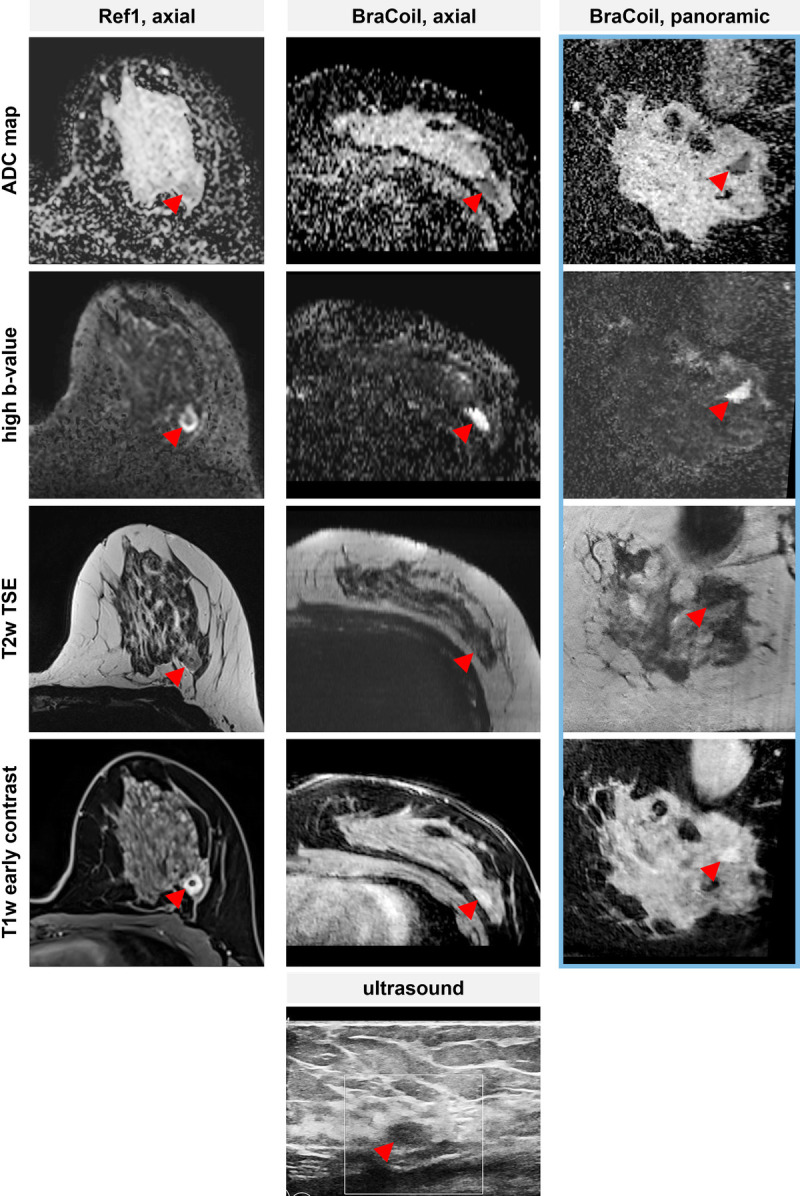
Clinical assessment case using the BraCoil in supine position. An exemplary case of clinical breast cancer assessment is presented. It highlights the advantages of supine imaging with the BraCoil. Because of the matching breast geometry between the panoramic breast MRI with the BraCoil and US, the lesion could be identified and biopsied in second-look US. The apparent diffusion coefficient (ADC) map (first row) shows low values of 1.18 × 10–6 mm^2^/s in the mass lesion at 2:00 in the left breast. Calculated high b-value (b = 1400 s/mm^2^) images (second row) show a corresponding hyperintensity of the lesion, while appearing slightly hyperintense on the T2-weighted turbo spin echo images (third row). The early T1-weighted image with fat suppression after contrast agent application (fourth row) shows a noncircumscribed mass lesion with distinct and heterogeneous enhancement and plateau curve type. The lesion was assigned a Kaiser Score^57^ of 5 and a BI-RADS 4 category and subsequently underwent ultrasound-guided biopsy, and was diagnosed as tubular adenoma. The targeted ultrasound examination (bottom row) showed a hypoechoic noncircumscribed mass corresponding to the MRI. Ultrasound guided 14G-core biopsy revealed invasive breast cancer. All MR images were acquired covering both breasts, for display purposes only the left breast with the lesion is shown. Images with the “16-channel Sentinelle Breast Coil” (Ref1) were acquired after biopsy and placement of a localization clip.
